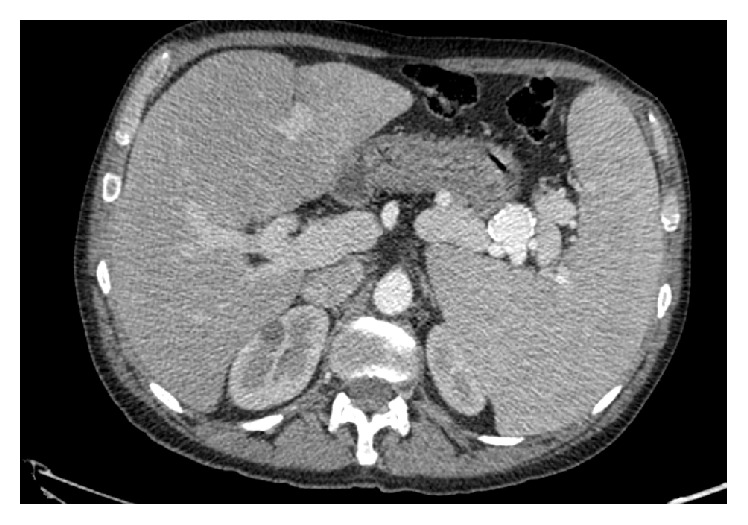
CT scan of the abdomen using IV contrast, performed before aneurysm embolization. A markedly enlarged spleen and a saccular aneurysm of the splenic artery with calcified walls are seen. A significant portal vein dilation with an increased splenoportal axis calibre is also present.
